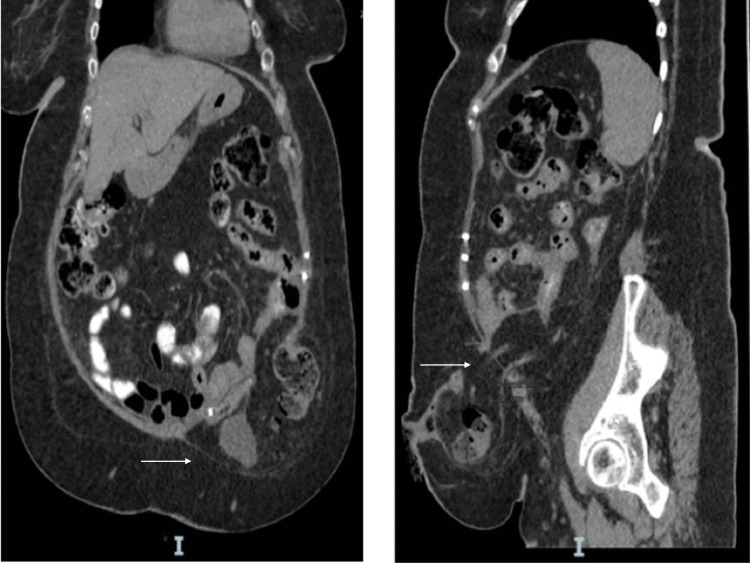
Follow-up CT abdomen and pelvis scan demonstrating recurrent parastomal hernia containing distal colon and omental fat six months post-initial laparoscopic Sugarbaker repair.
